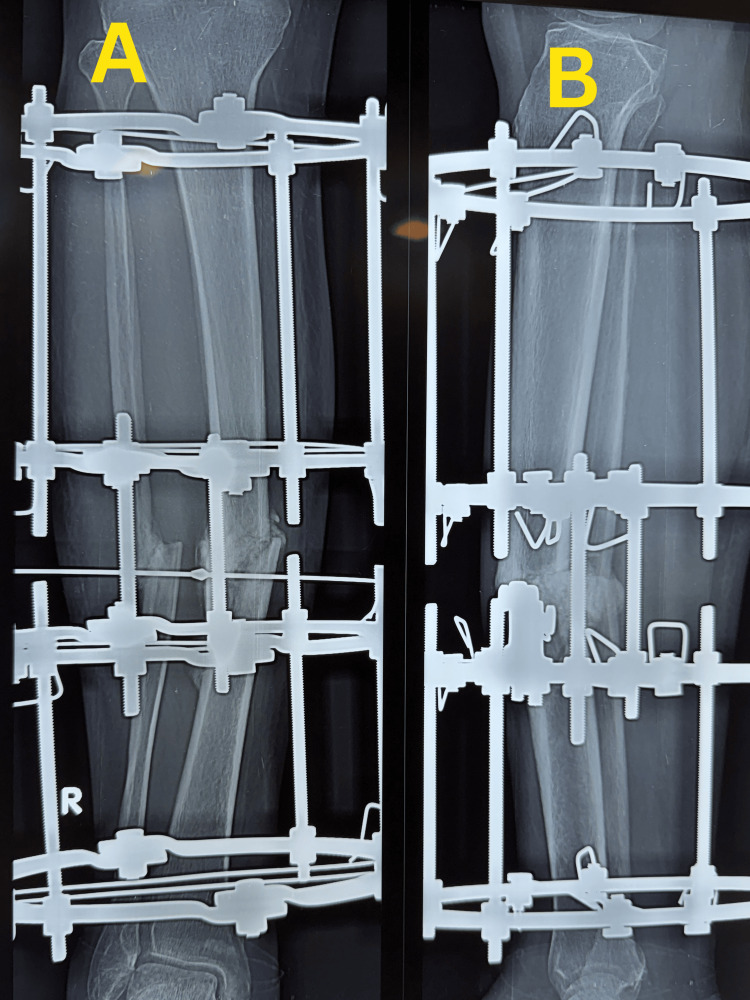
Follow-up radiographs demonstrating fracture union with maintained alignment Radiological union of the midshaft right tibial fracture is demonstrated by bridging callus across at least three cortices, with maintained alignment. (A) Anteroposterior view. (B) Lateral view.
